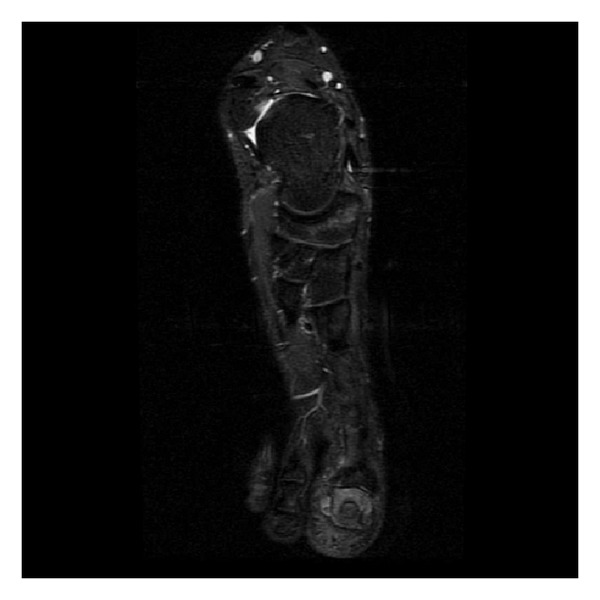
Preoperative coronal T1-weighted MR image of the left foot.
